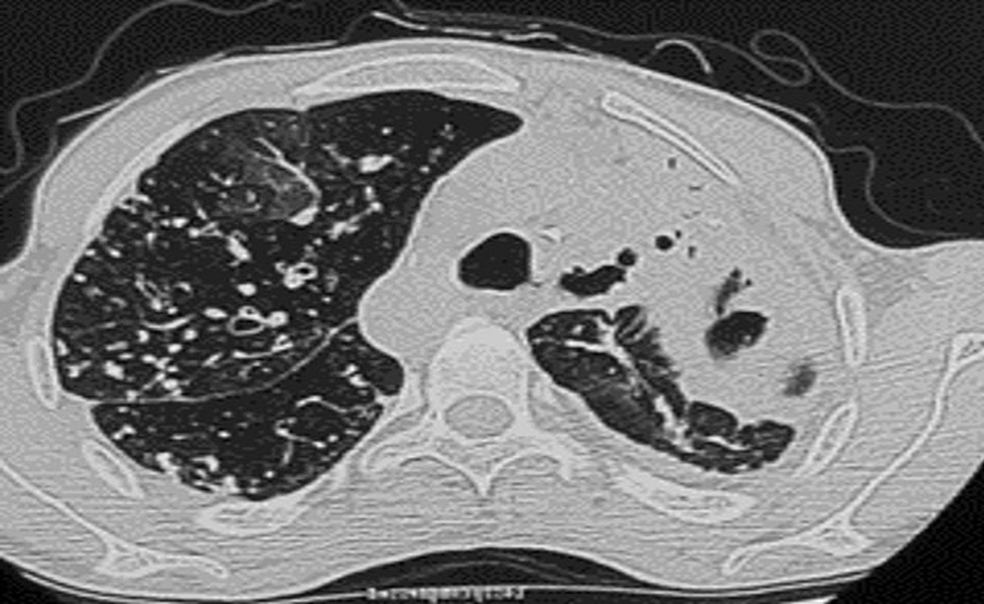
Chest CT showing left upper lobe collapse consolidation along with cavitation.
